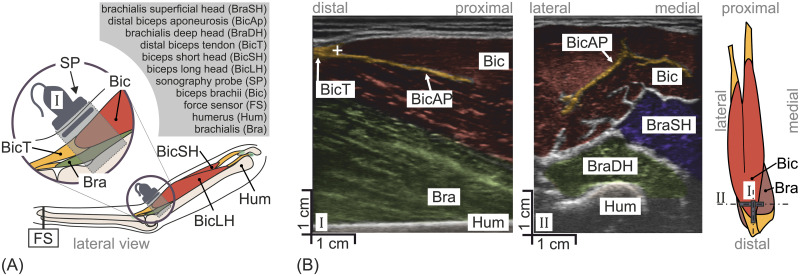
In (A) the lateral view of the experimental setup in form of an anatomical sketch is shown. An ultrasonic probe (SP) is used to image a cross-section of the distal part of the upper arm. The cross-section contains the distal tendon of the *biceps brachii* (BicT). Simultaneously, the force generated by the arm is measured with a force sensor (FS) near the wrist (cmp. Fig 3). The *biceps brachii* (Bic), its long head (BicLH), its short head (BicSH), *brachialis* (Bra) and *humerus* (Hum) are also labeled. In (B) the ultrasonic images of two orthogonal cross-sections are depicted. The orientation of both imaging planes are marked in a sketch on the right side, which shows the anterior perspective on the Bic and Bra. Orientation I was the only imaging plane used during the presented study (cmp. (A)). The structures of the Bic are marked in red. The junction of Bic and BicT was observable in this view (white cross) as well as part of the *aponeurosis* (BicAP). Imaging plane II is orthogonal to imaging plane I. The furcation (wing-like structure) of the *aponeurosis* was observable (marked in orange) as well as different parts of the Bra muscle group—the *brachialis deep head* (BraDH) in green and the *brachialis* superficial head (BraSH) in blue.
